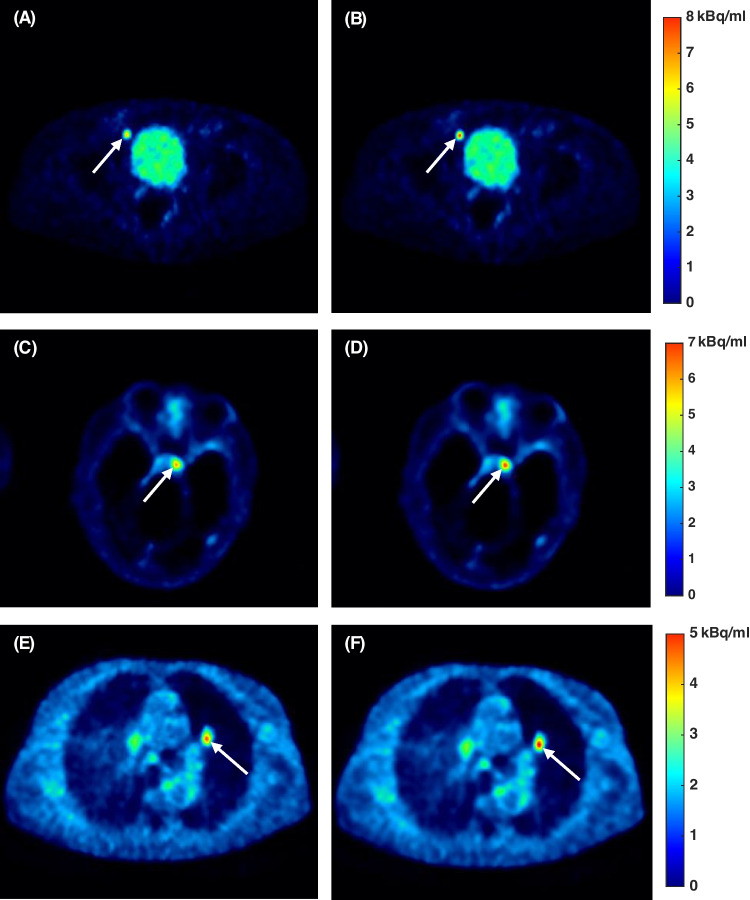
Image comparison of patient data. Soft tissue lesion (lymph node) in [^68^Ga]Ga-PSMA PET: (A) non-PRC, (B) PRC. Bone lesion in [^68^Ga]Ga-DOTATOC PET: (C) non-PRC, (D) PRC. Lung lesion in [^68^Ga]Ga-DOTATOC PET: (E) non-PRC, (F) PRC
